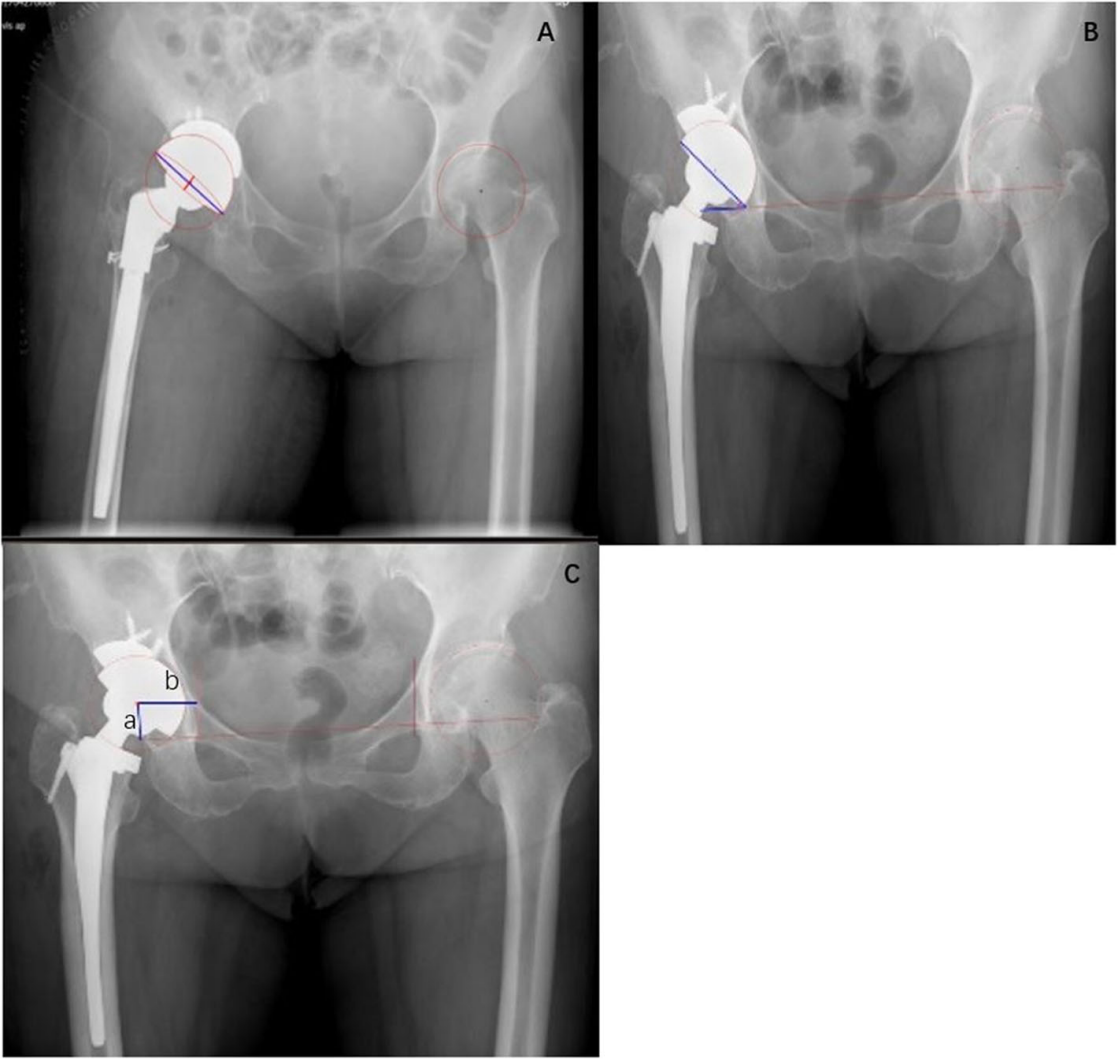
Measurement with anteroposterior view of an X-ray. a measures the angle of anteversion, anteversion angle = arcsin (D1/D2); D1: short red line of the ellipse; D2: long blue line. b measures the angle of abduction. c shows the vertical and horizontal position of COR. A: vertical position of COR; B: horizontal position of COR
